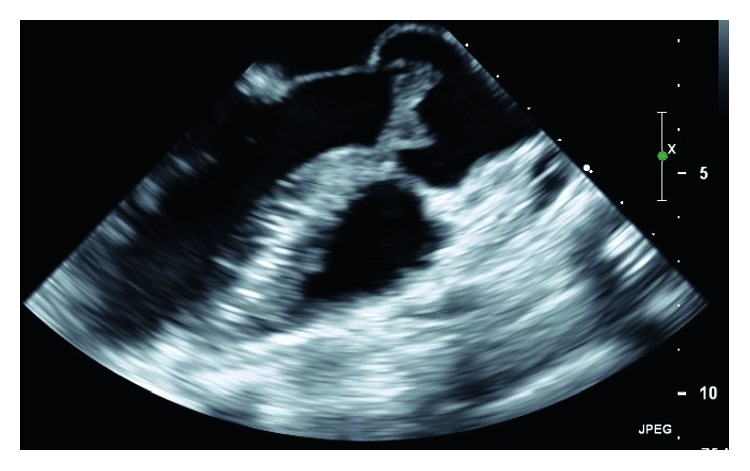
Initial echocardiogram showing a 2 cm mass on the right and noncoronary cusps of the aortic valve.
